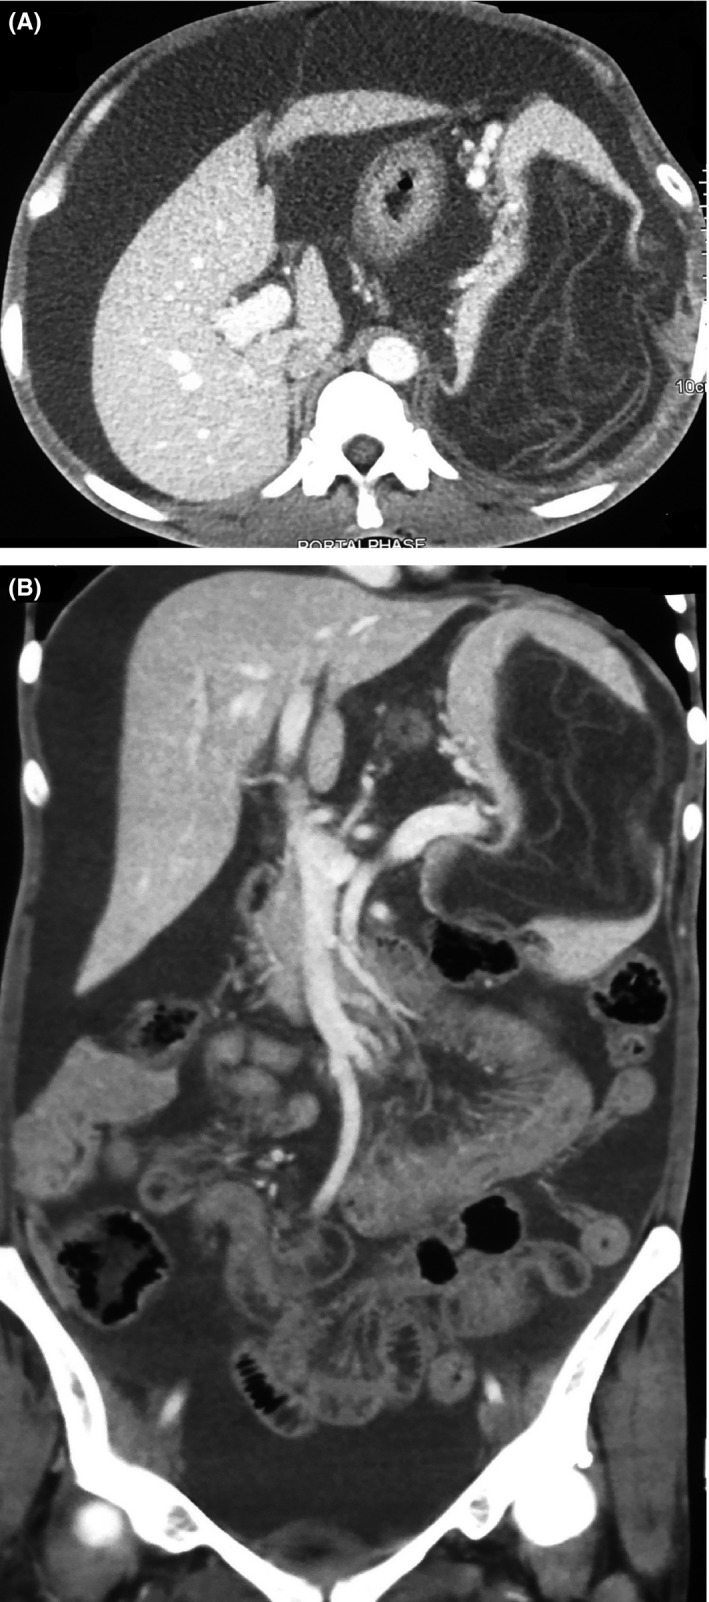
CT finding suggesting gross ascites and nonenhancing hypodense cystic lesion within splenic parenchyma with floating membrane within; feature suggestive of ruptured hydatid cyst (A,B)
